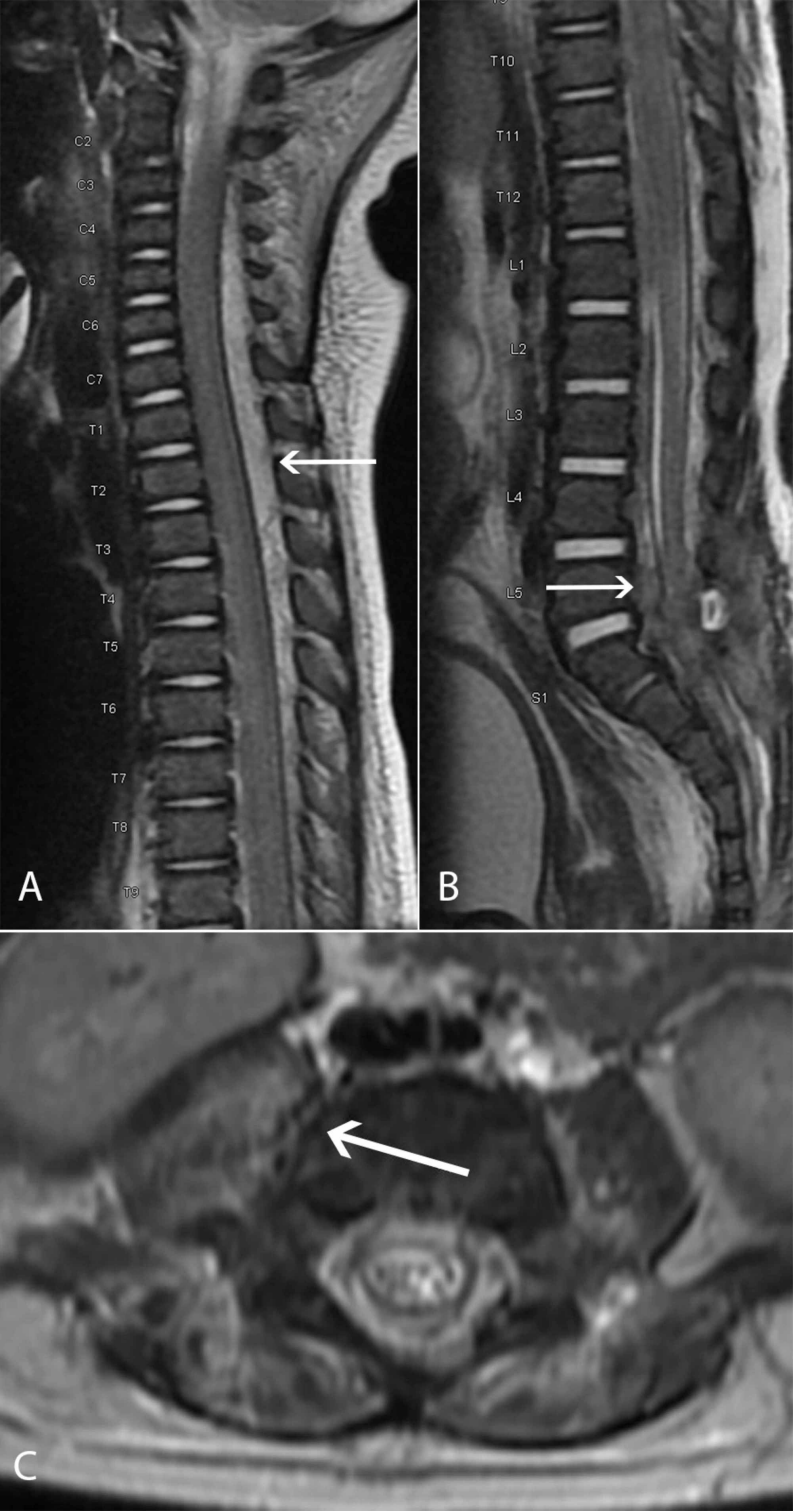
T2 MRI of the spine showing a significant epidural abscess extending from C2 to the sacrum, with cervicothoracic spinal cord compression (A), lumbosacral neural compression (B), and extension to the psoas/presacral musculature (C). Arrows demonstrate the epidural (A and B) and psoas abscesses (C).
